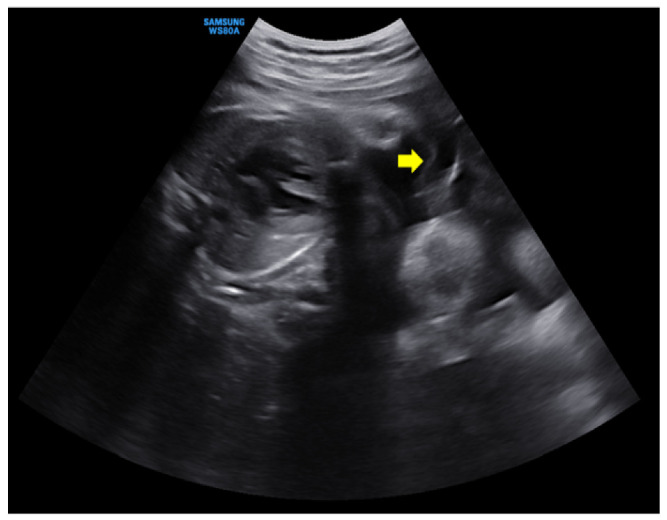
An ultrasonographic examination at 6 days before visiting emergency room. The intertwine membrane (yellow arrow) was well seen, and the amniotic fluid of both fetuses was normal.
